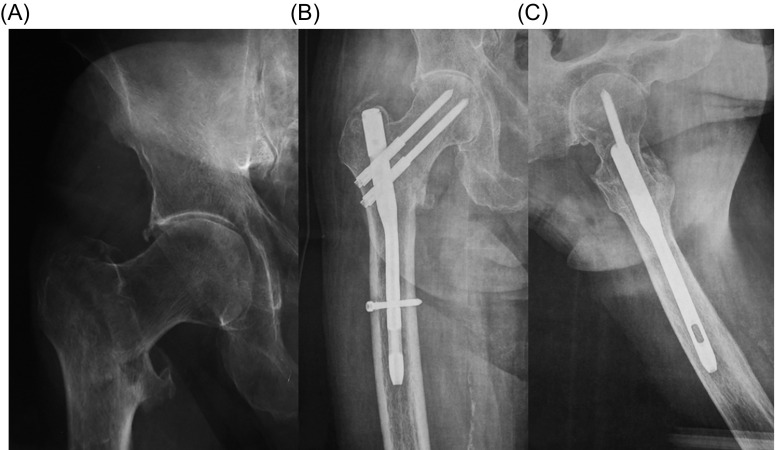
(A) Anteroposterior radiograph of the right hip of a 75-year-old man shows an AO/OTA-31-A1 fracture. (B) Anteroposterior and (C) lateral radiographs of the right hip nine months after surgical treatment show fracture healing. The patient was alive with excellent function.
